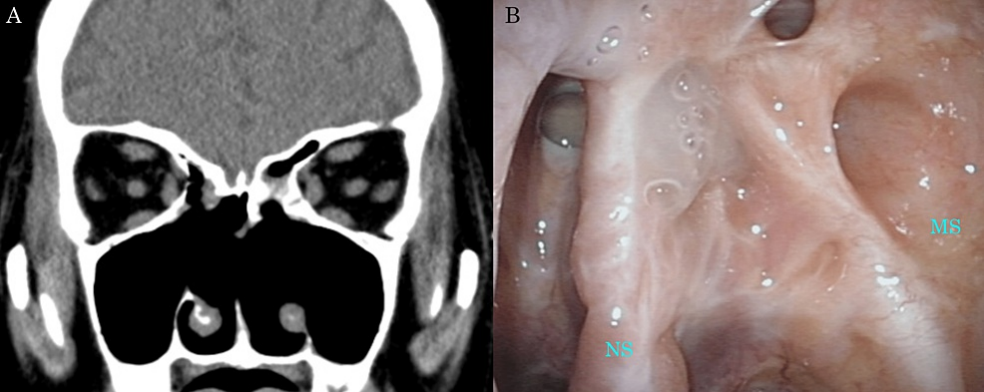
Images of sinus CT and nasal endoscopy one year after surgery (A) Sinus CT and (B) nasal endoscopy revealed no recurrence of FRS. CT: computed tomography, FRS: fungal rhinosinusitis, MS: maxillary sinus, NS: nasal septum
